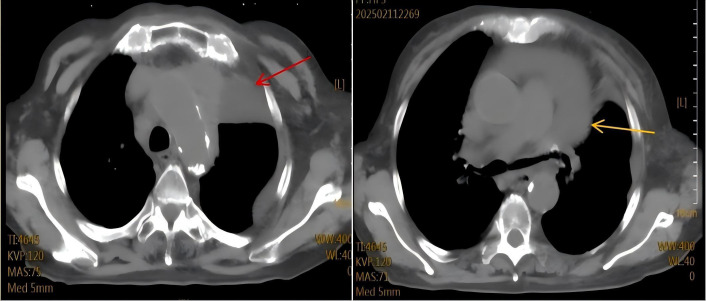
Disease progression upon readmission in february 2025.Chest CT scan demonstrates enlargement of the mediastinal tumor (red arrow), significant narrowing of the left main bronchus, and a newly developed large pericardial effusion (yellow arrow).
